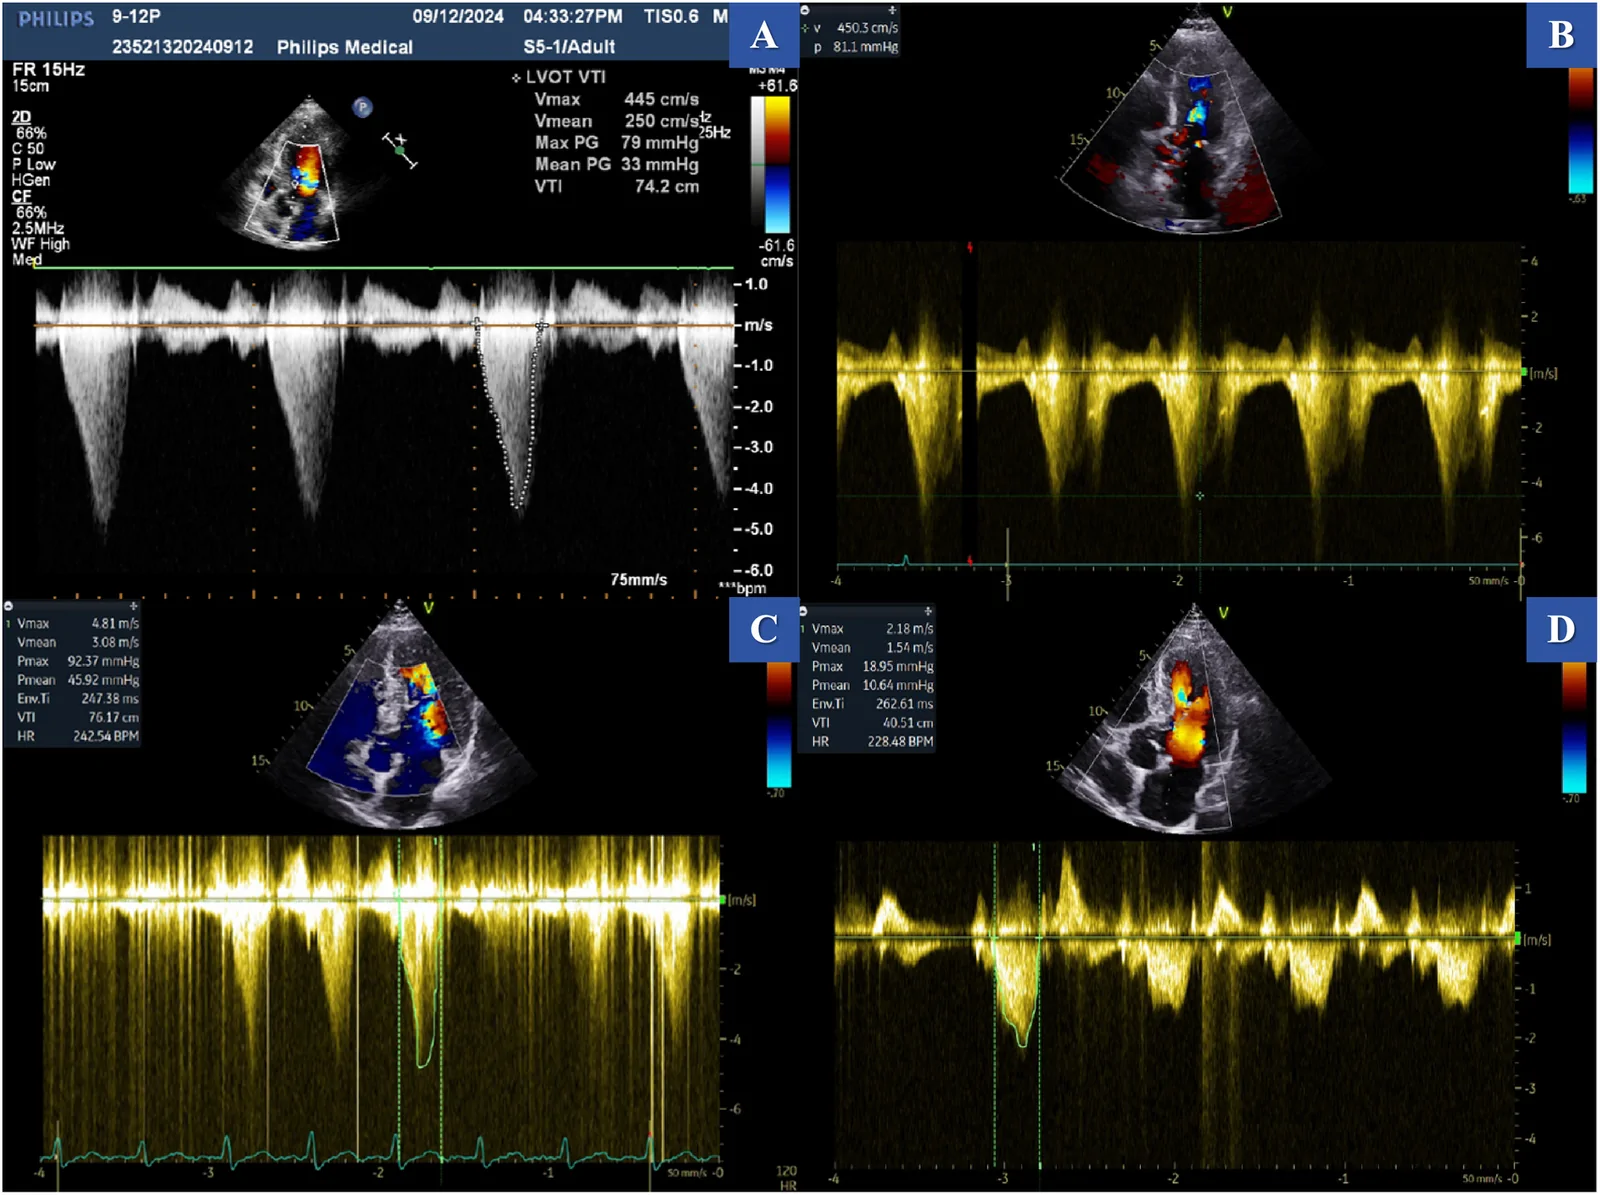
Echocardiography findings of asymmetrical septal hypertrophy with left ventricular outflow tract stenosis or obstruction (A,B), and stress echocardiography showing latent obstruction (C,D).
